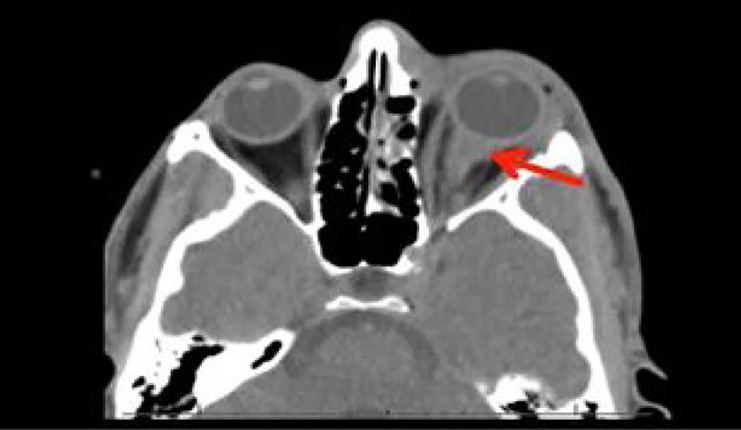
Computed tomography maxillofacial without contrast demonstrating an intraconal hematoma (red arrow), thickening of the optic nerve with proptosis
